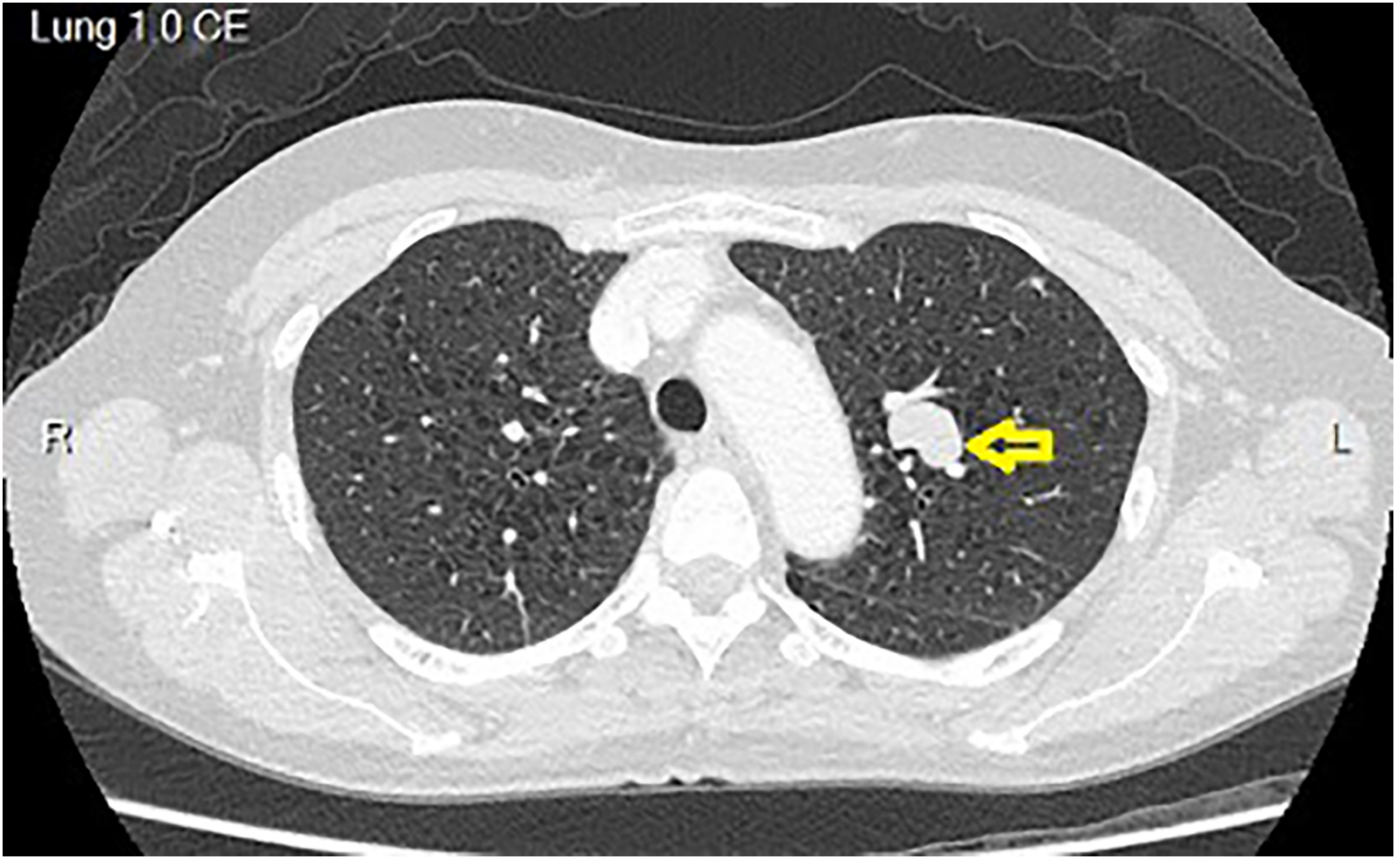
CT images revealed a solitary nodule with a diameter of 18.9 × 17.8 mm in the left upper lobe. The tumor is located in the hilum area. Besides, the CT images showed severe emphysematous change over bilateral lung parenchyma. CT, computed tomography.
